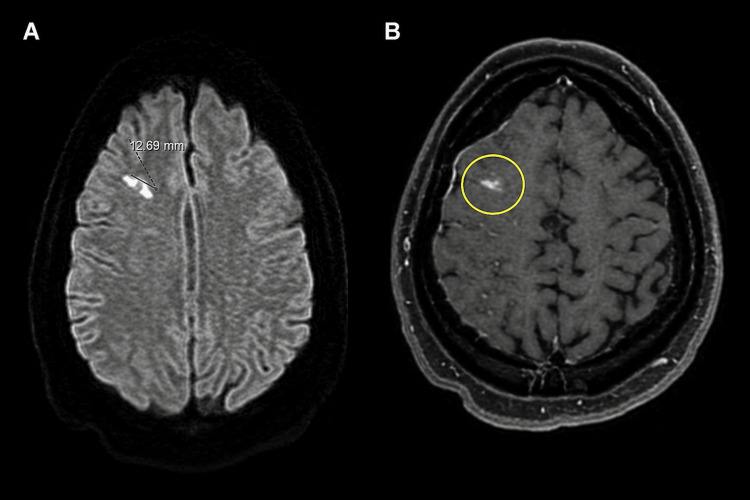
Multimodal MRI demonstrating right frontal lobe lesion with restricted diffusion and post-contrast enhancement A) Axial diffusion-weighted imaging (DWI) of the brain demonstrates hyperintense lesions within the right frontal lobe, with corresponding hypointensity on the apparent diffusion coefficient (ADC) map (not shown), consistent with restricted diffusion. These findings correlate with the hypodensity observed on CT in Figure 1; B) The circled lesion demonstrates enhancement on a CUBE multiplanar reformatted (MPR) sequence, a high-resolution three-dimensional fast spin-echo MRI technique. This finding indicates disruption of the blood–brain barrier and corresponds to the region of restricted diffusion seen on DWI/ADC (Figure 3A). The imaging appearance supports an active process, such as an inflammatory or demyelinating etiology, in the appropriate clinical context.
